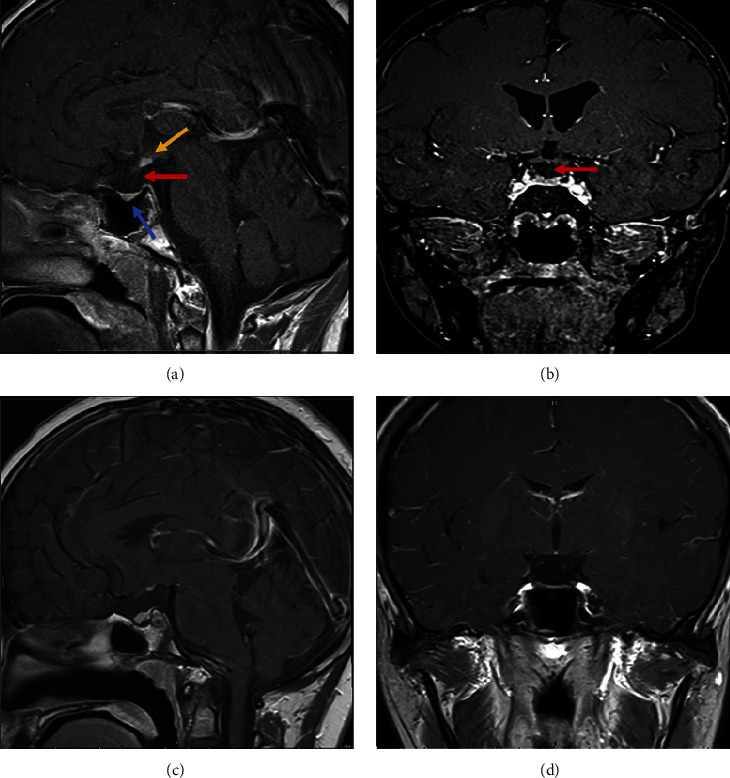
Pituitary magnetic resonance imaging (enhanced T1-weighted images). (a) The sagittal slice showed a thin pituitary stalk (red arrow), an ectopic posterior pituitary (yellow arrow), and anterior pituitary hypoplasia (blue arrow). (b) The coronal slice showed a thin pituitary stalk (red arrow). (c) The sagittal slice showed the absent pituitary stalk. (d) The coronal slice showed the absent pituitary stalk.
